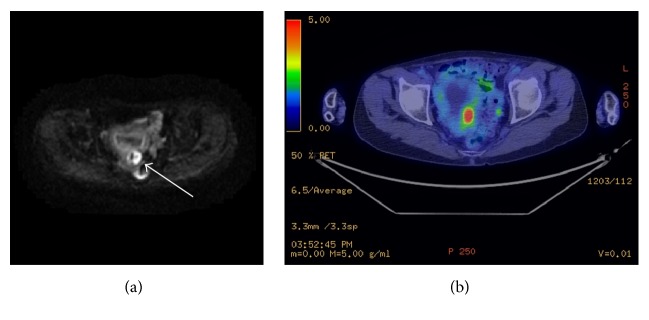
(a) Diffusion weighted magnetic resonance imaging. The abnormal signal with a diameter of approximately 2 cm on the left sidewall of the uterine corpus (at indicated by arrow). (b) FDG-PET. Abnormal uptake in the tumor had a maximal standardized uptake value (SUV (max)) of 7.79.
